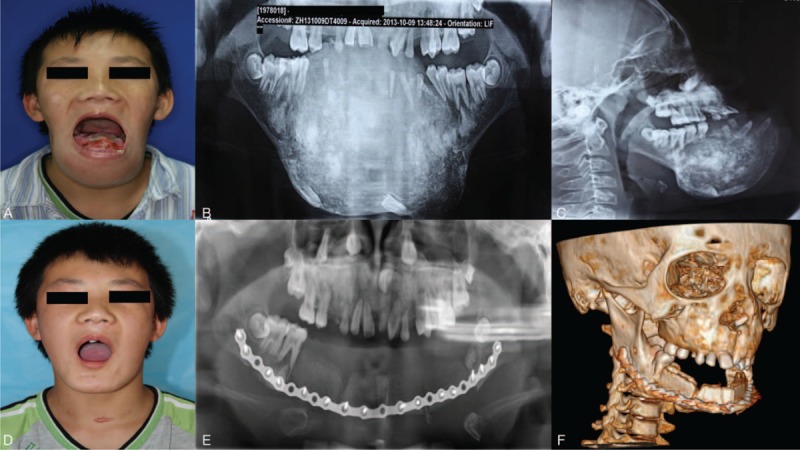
(A) The adolescent patient with a huge mass extending along the mandible body. (B) Preoperative panorex X-ray showed a characteristic radiographic feature of familial gigantiform cementoma (FGC) with well-circumscribed radiopaque areas involving all quadrants of the jaw, with mandible being more severely damaged. (C) Lateral view of FGC. (D) Postoperative view of patient after bilateral mandibulectomy. (E) Postoperative panorex X-ray showed vascularized iliac bone flap for reconstruction. (F) 3-D computed tomography reconstruction of postoperative view.
